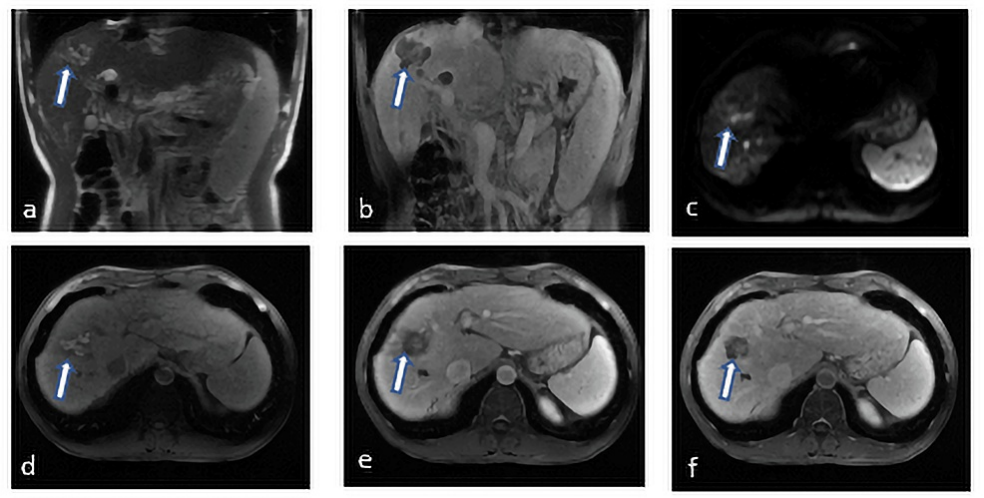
Hepatocellular adenoma in a 47-year-old female. (A) Coronal T2-weighted SSFSE BH image shows hyperintense lesion (arrow) segment 8, (B) coronal T1-weighted 3D AX GRE image shows hypointensity lesion (arrow), (C) diffusion-weighted images show that the lesion is hyperintense (arrow), (D) the lesion (arrow) is hypervascular on arterial phase (E) with washout on portal-venous phase, and (F) on hepatobiliary phase the nodule (arrow) is hypointense with surrounding liver parenchyma. 3D AX GRE: three-dimensional axial gradient; SSFSE BH: single-shot fast spin echo black blood
